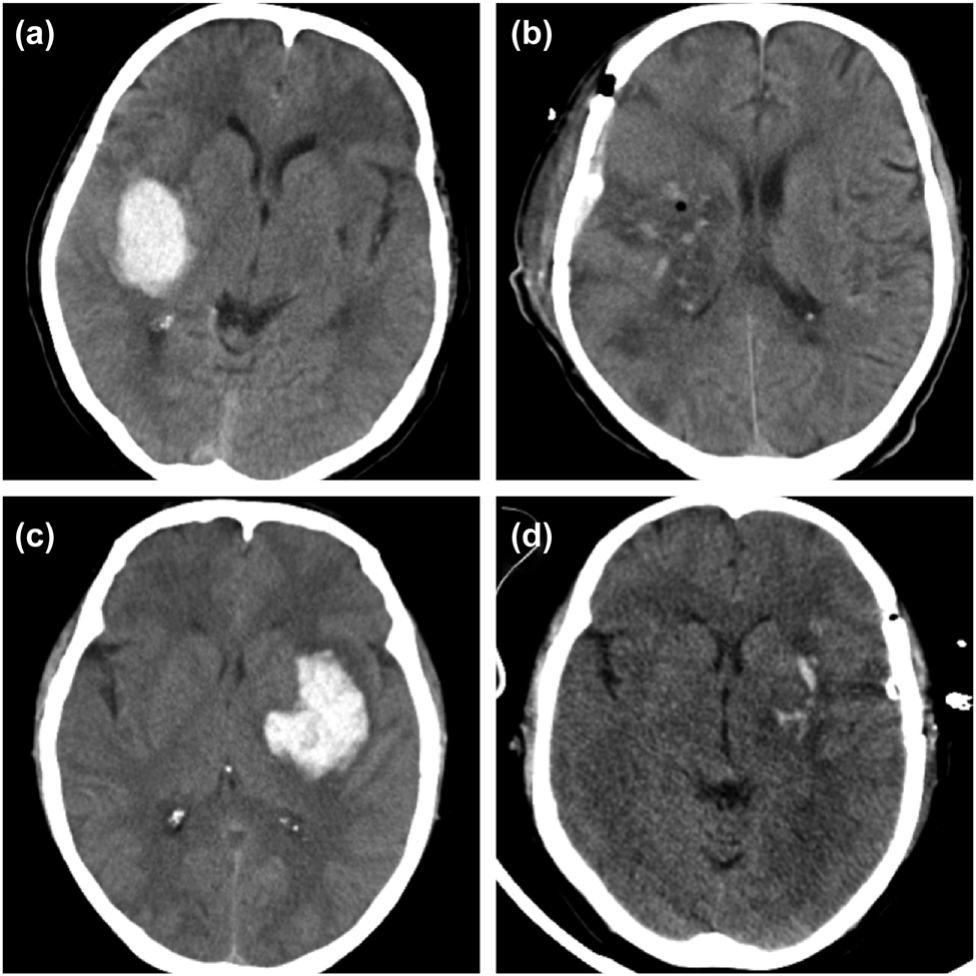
(a and b) Preoperative CT and postoperative CT of case 1 with a right basal ganglia hematoma of 27.4 ml, he received a hematoma evacuation through a transsylvian-transinsular approach 5.2 h from ictus. (c and d) Preoperative CT and postoperative CT representing a left basal ganglia hematoma of 32.8 ml operated through a transsylvian-transinsular approach 4.9 h from ictus.
